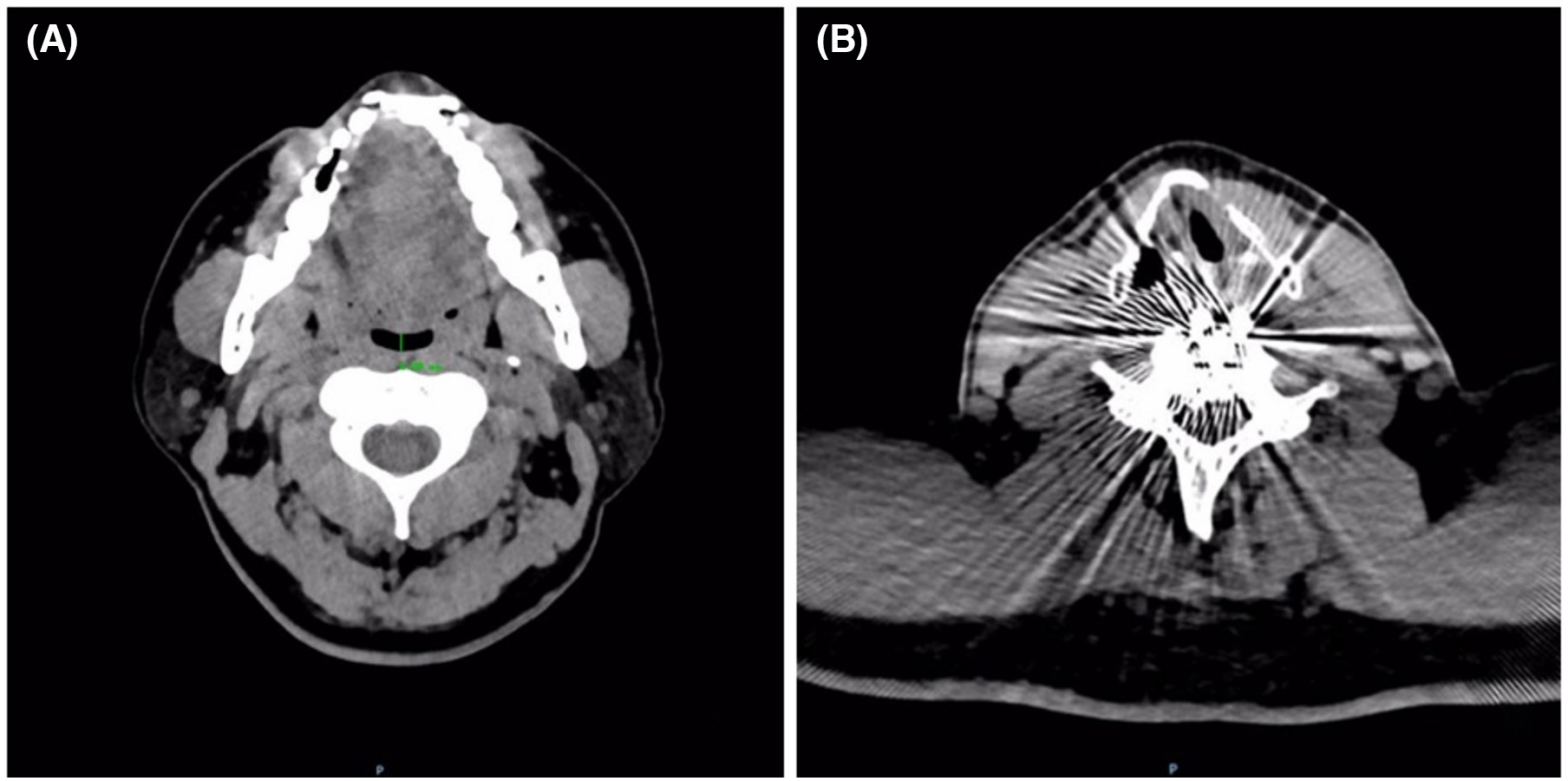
The upper airway computed tomography (UACT) of Case 1. (A) Narrowing of the sagittal diameters (the narrowest point, approx. 4.88 mm) of the oro‐pharyngeal region; according to the data available in China,^6^ the sagittal diameters of this region in the OSA‐free middle‐aged male population is about 13 mm. (B) Post‐operative changes (the layer of glottis) in the cervical spine are shown.
